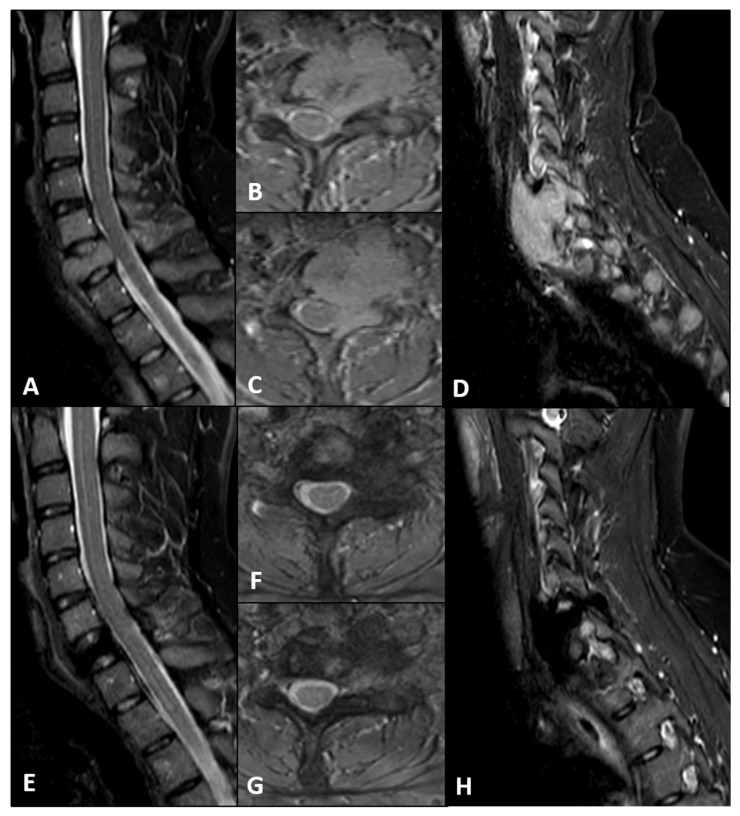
MRI radiographic evolution under Neoadjuvant Denosumab Therapy. (A–D) Baseline assessment. T2-weighted Gradient Echo (GRE) Sagittal (A,D) and Axial (B,C) sequences showing a hyperintense, expansile mass involving the C7 vertebra with significant paravertebral extension. (E–H) Twelve-month follow-up. Corresponding T2-weighted GRE Sagittal (E,H) and Axial (F,G) views following Denosumab treatment. Comparison: The follow-up images demonstrate a reduction in tumor volume (shrinkage) and a signal hypointensity consistent with matrix ossification. The sagittal views (E,H) further document the progression of the local kyphotic deformity.
